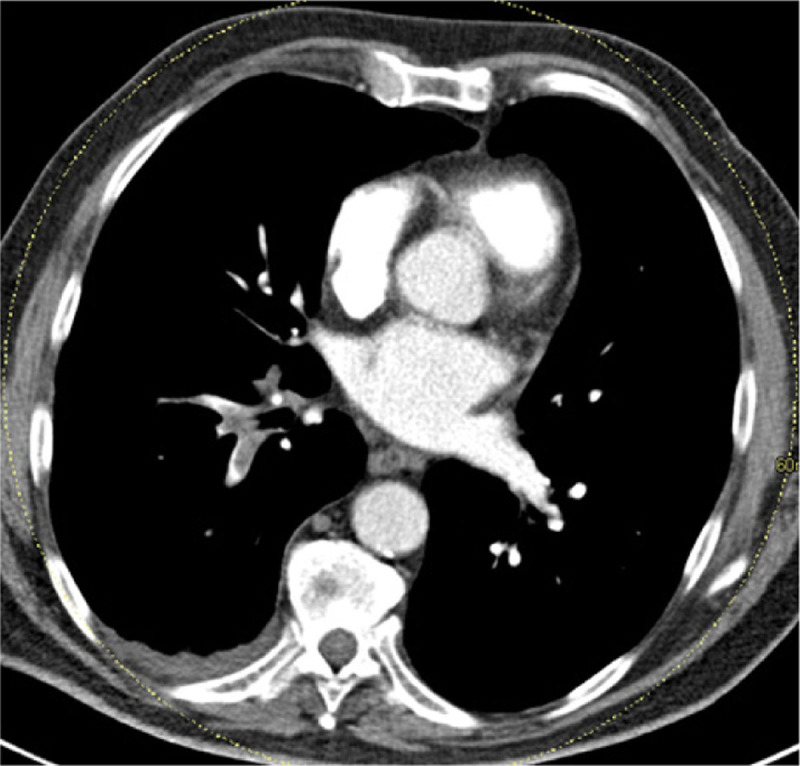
CT angiography with pathognomonic arterial filling defects.
